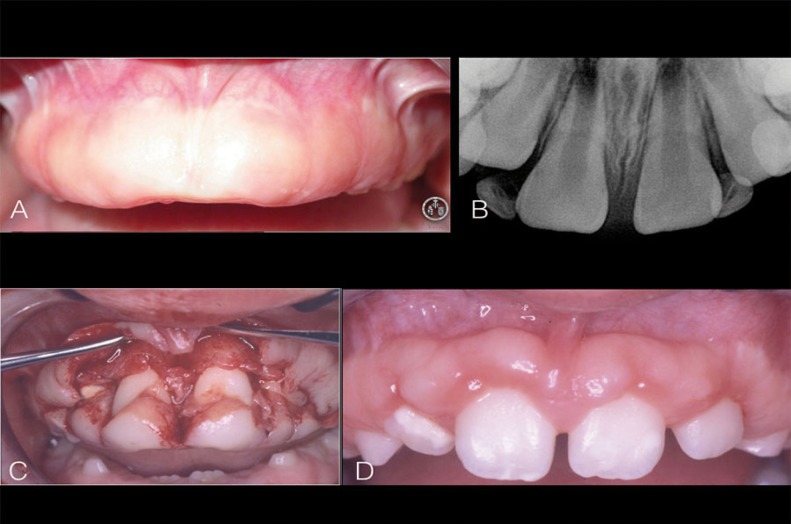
Case 1: A) intraoral view; B) Occlusal radiograph; C) Intrasurgical view of infernal bedel incisión gingivectomy prior to eliminación of excess tissue; D) Intraoral view 6 months after surgery.
